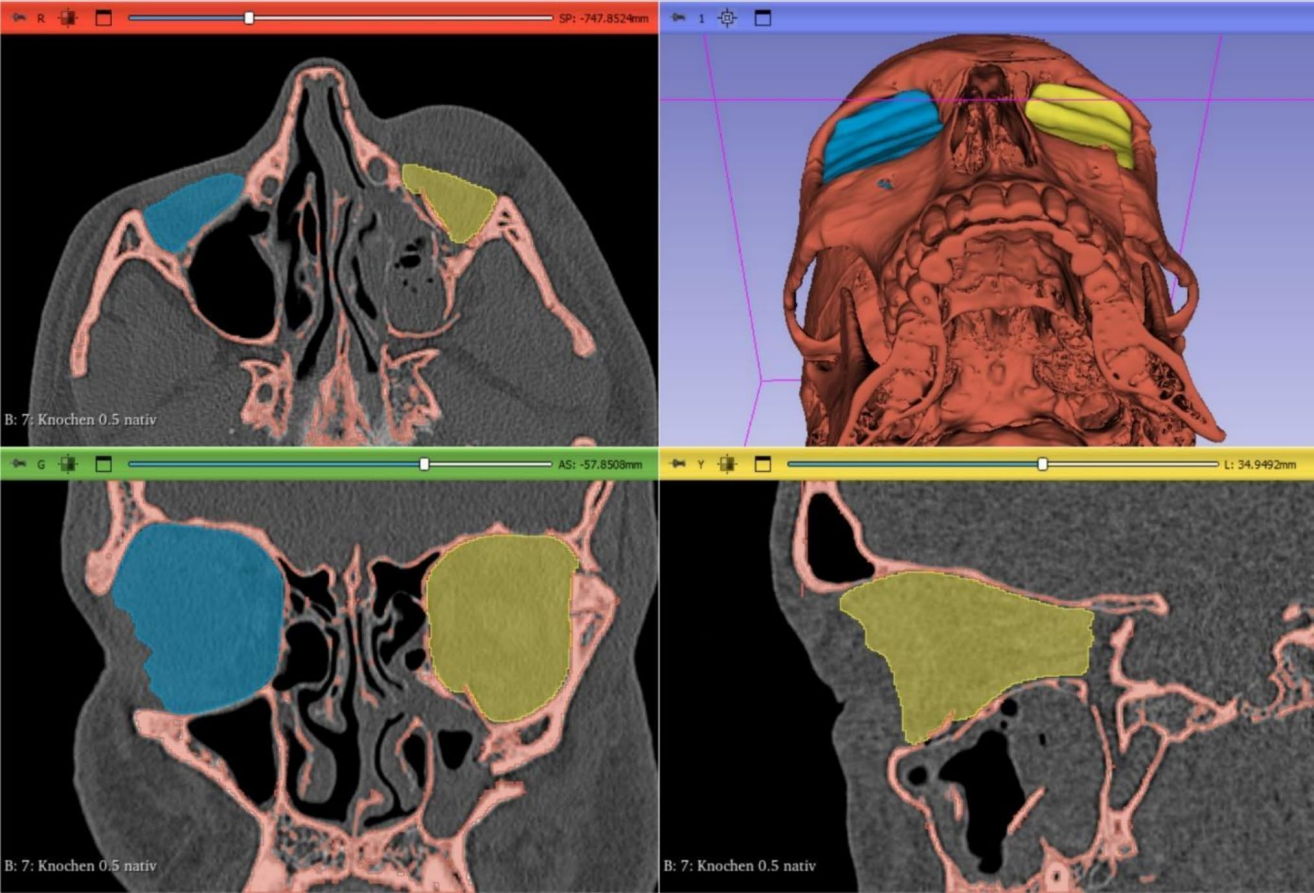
Preoperative CT scan of the midface in axial (A), coronal (C), sagittal (D) and 3D reconstruction (B). Segmented volumes of fractured (yellow) and intact orbit (blue) were generated using 3D-Slicer software
